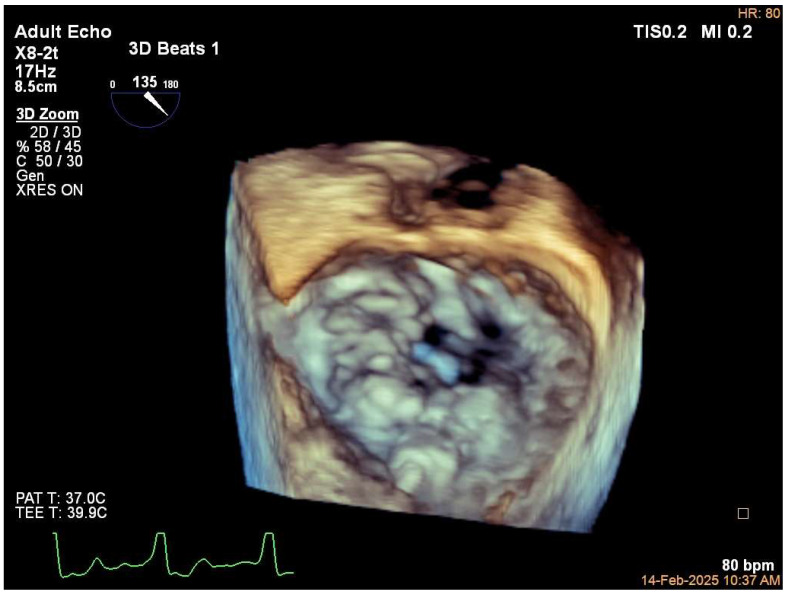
Three-dimensional transesophageal echocardiographic en face “surgical view” visualizing the atrial aspect of the mitral valve in a patient with significant mitral annular calcification (MAC), showing a more pronounced calcification of the posterior mitral annulus.
